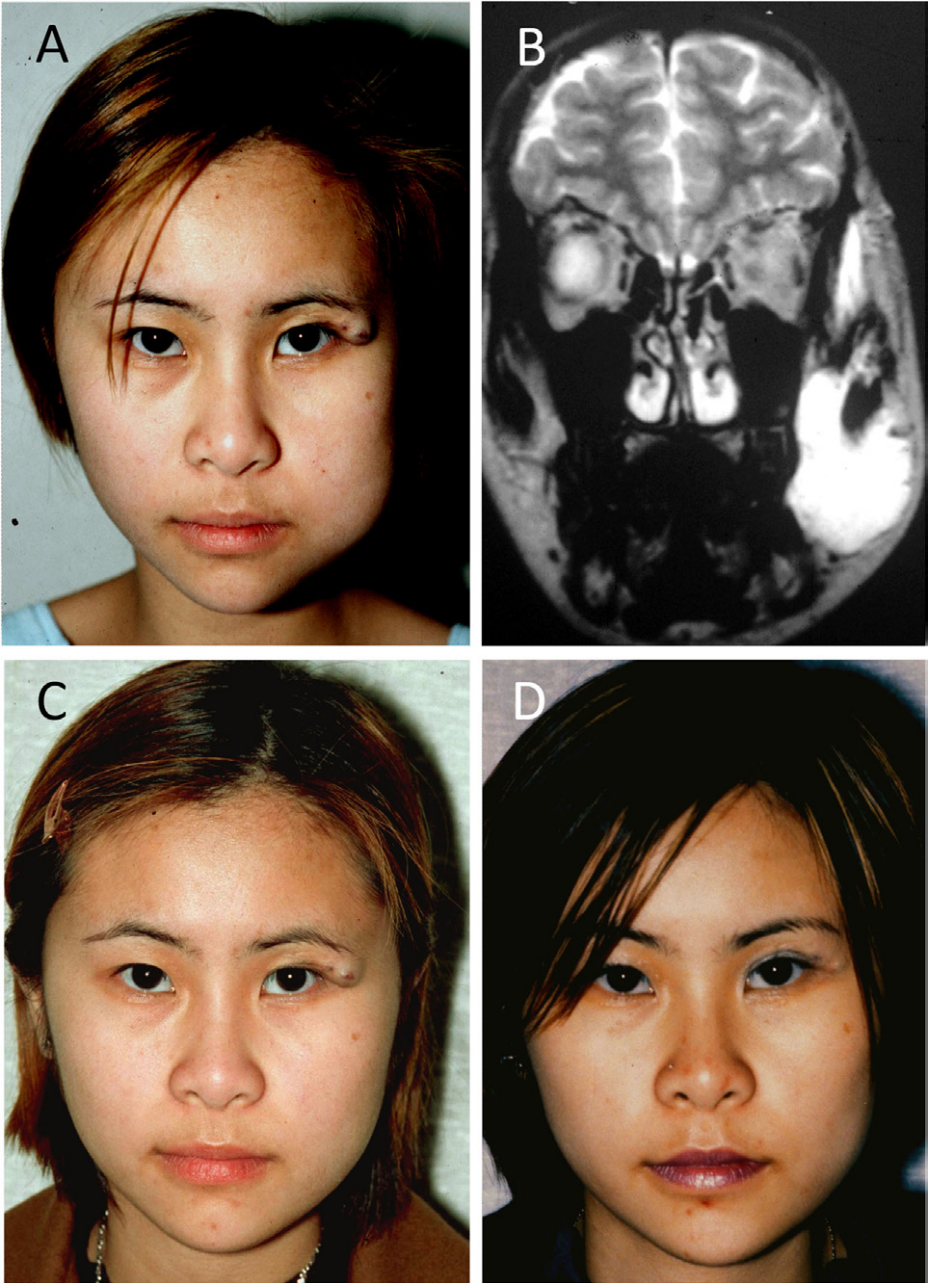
A 15-year-old female with a venous malformation (VM) affecting her left cheek and peri-orbital area (A). A T2-weighted magnetic resonance imaging showing the VM within the left buccal space, extending into the temporal region, deep to the temporalis muscle (B). Ethanol sclerotherapy of the VM led to improved facial symmetry (C). The residual VM over the lateral canthus was debulked and remained unchanged over 2 years (D). Reproduced with permission from the Australian and New Zealand Journal of Surgery (27).
